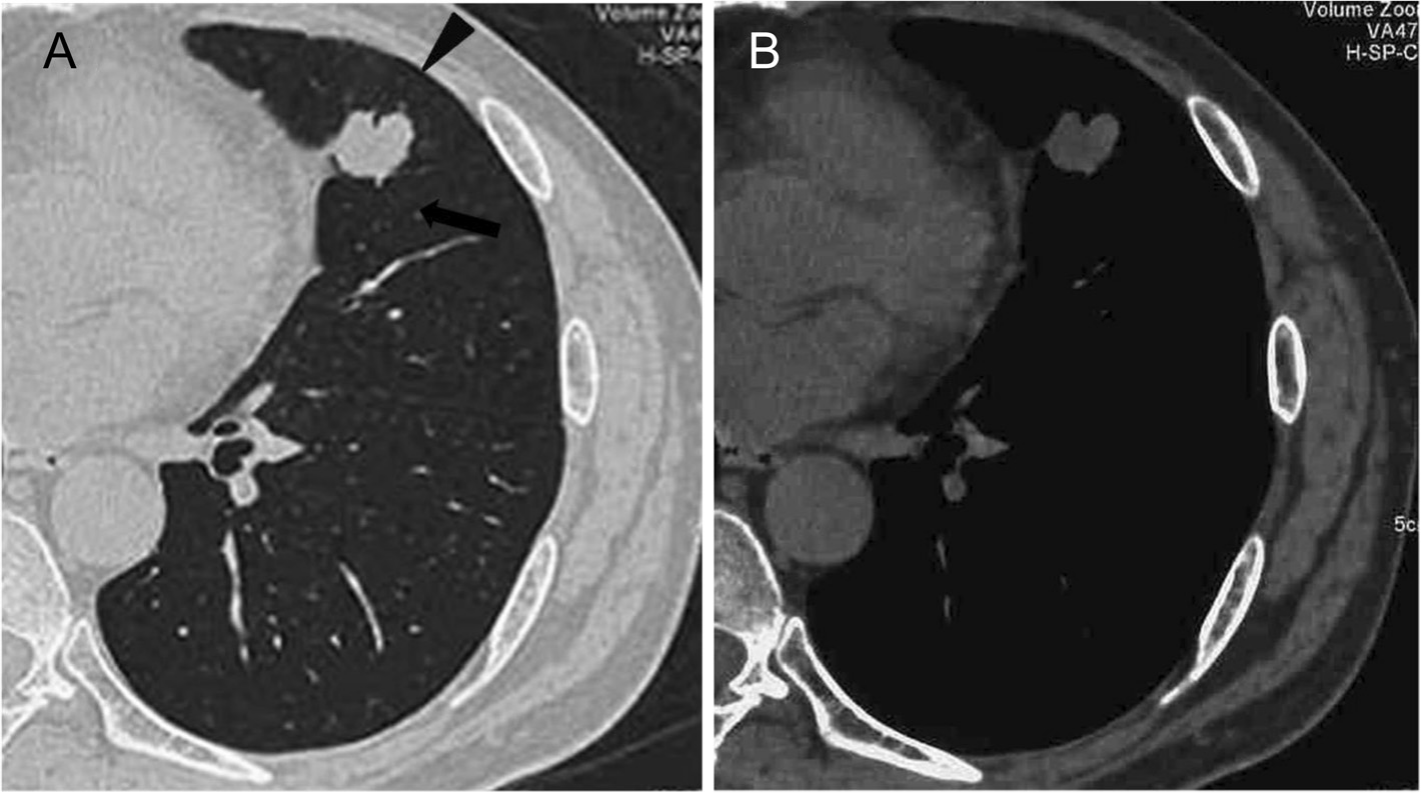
Plain computed tomographic image (lung window) showing the nodes with spicula (*arrowhead*), notch (*arrow*), and pleural indentation in the lingual segment of the left lung. There is no evidence of calcification. (A) Lung window and (B) mediastinal window.
